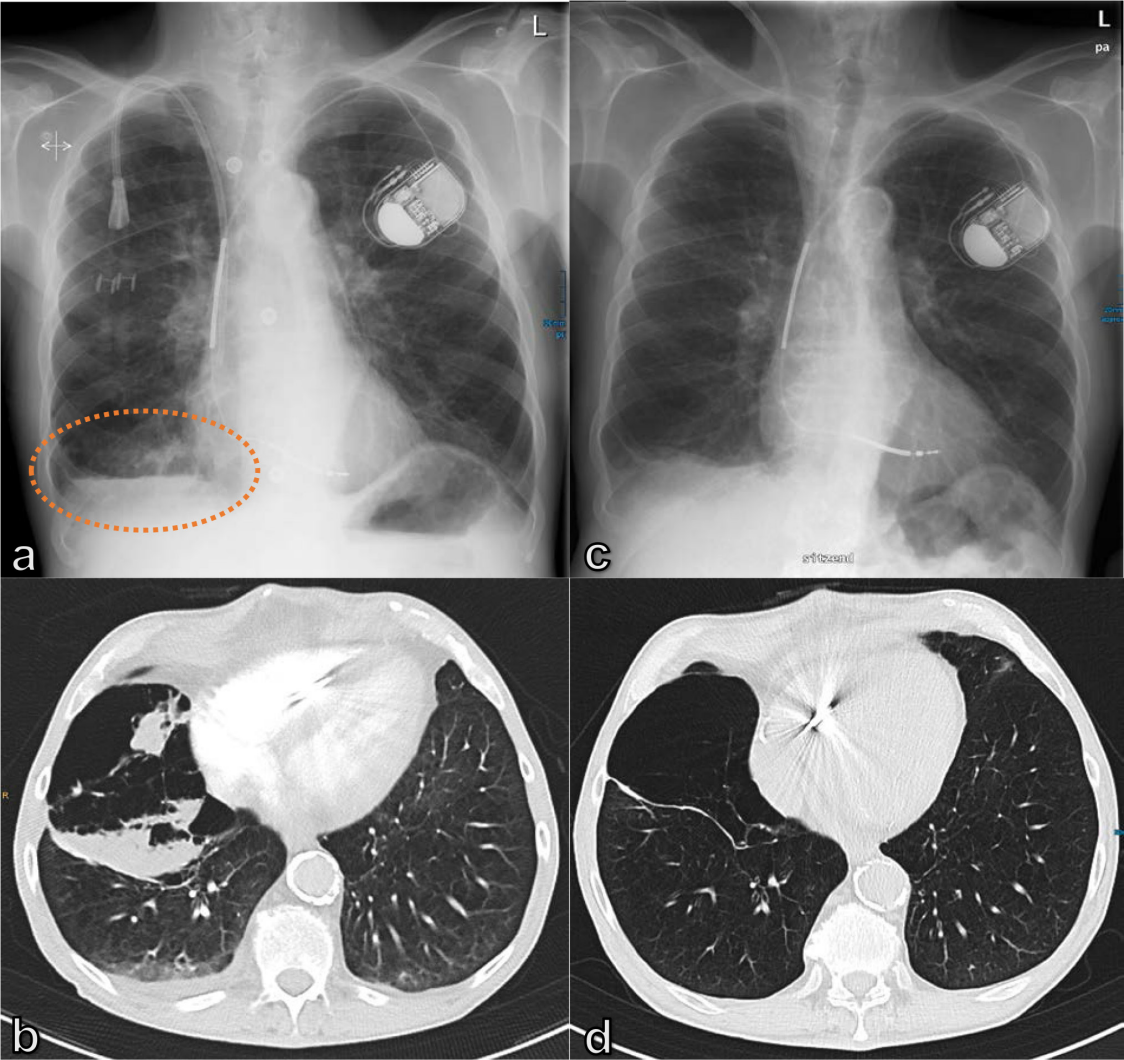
65-year-old male patient with COPD IV with massive aggravated dyspnea and tachypnea over the 3 days prior to admission: (a) CXR presenting a newly developed non-specific small opacity and gas-fluid level above the right diaphragm (circle), compared to an older image (c), while chest CT (b) depicts a newly demarcated consolidation in the dependent areas of the bullous parenchymal destruction of the middle lobe, as an inflammatory superinfection. (d) CT examination taken previously. COPD, chronic obstructive pulmonary disease; CXR, chest X-ray.
